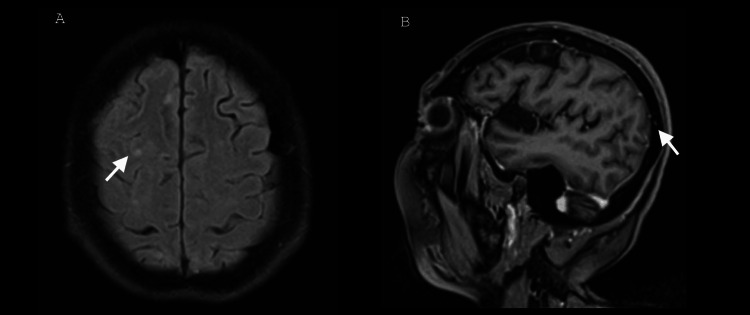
MRI of the patient in FLAIR and T1-weighted contrast-enhanced sequences, post-treatment. (A) Axial section at the cortical level of the frontoparietal lobe showing resolution of meningeal enhancement, with only residual ischemic microangiopathy (arrow). (B) Sagittal T1-weighted contrast-enhanced section showing absence of gadolinium uptake in the leptomeninges (arrow) of the right cerebral hemisphere. FLAIR: fluid-attenuated inversion recovery
